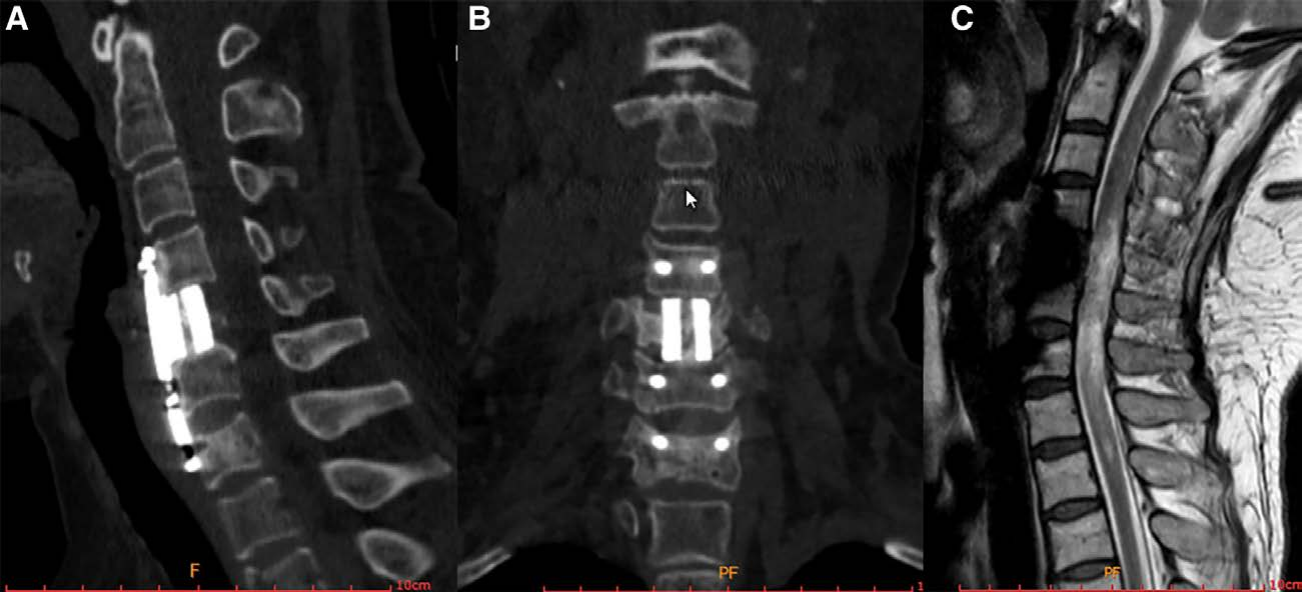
Post-operative CT and MRI scans at the six month of follow-up (a) lateral CT scan, (b) anterior CT scan, and (c) anterior MRI scan.
